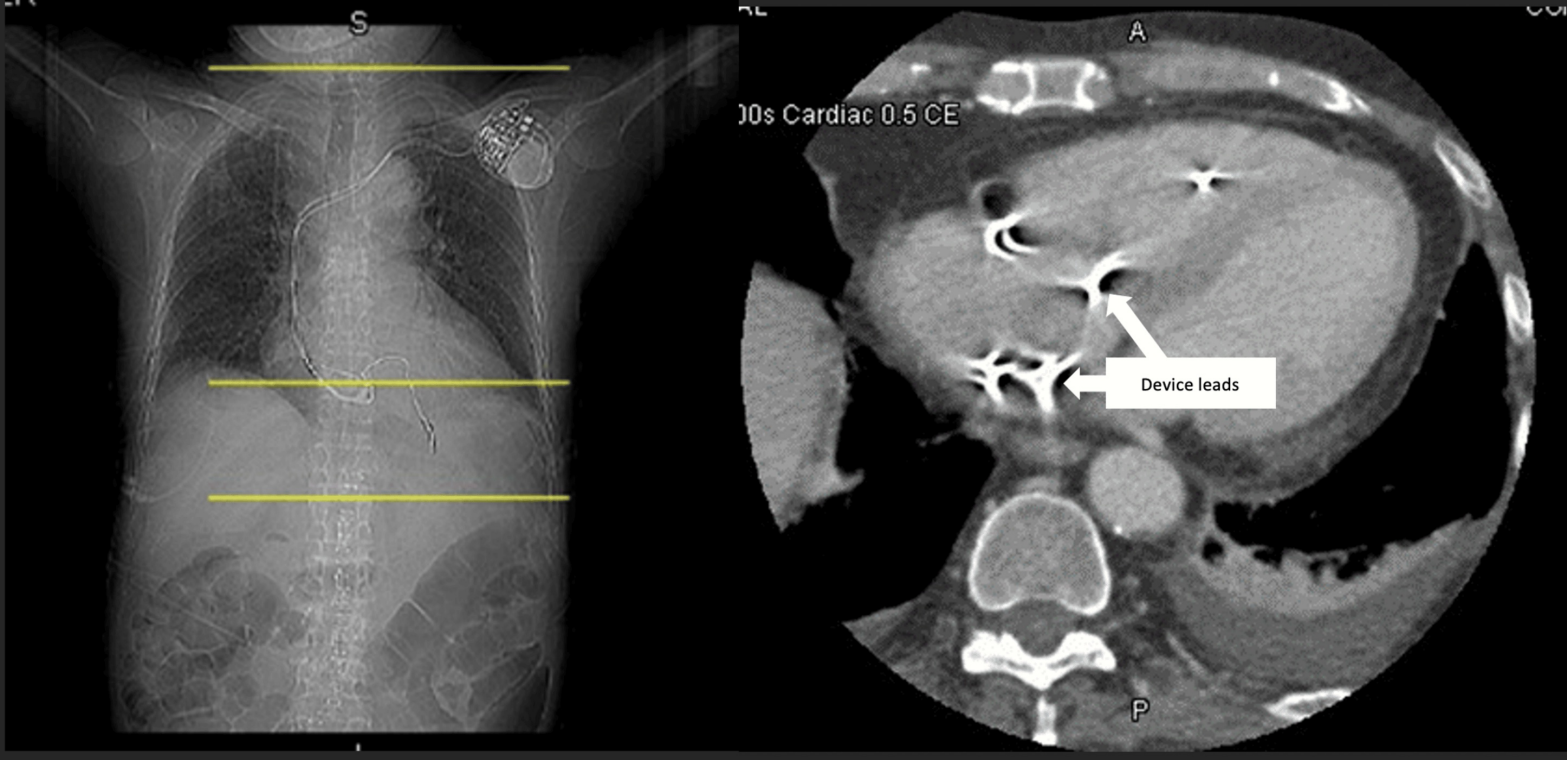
CT angio cardiac morphology 3D. Device leads seen as denoted by arrows. No specific abnormalities concerning for infection were seen.
